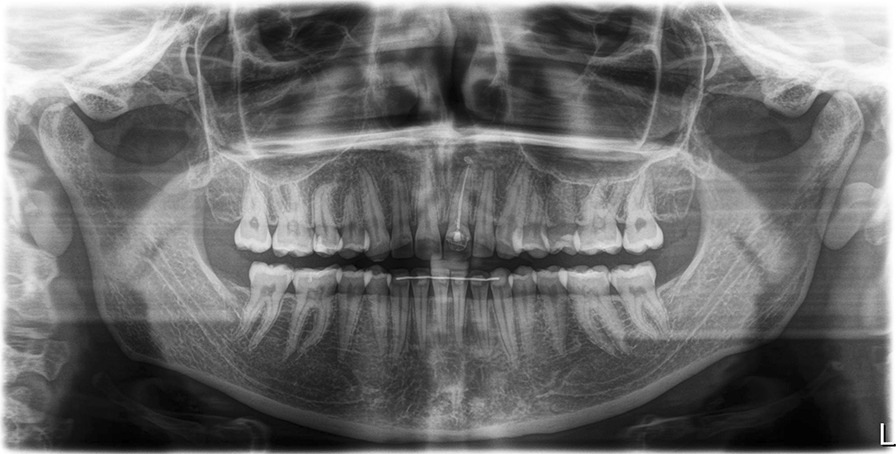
Panoramic radiograph of the patient showing symmetric but flattened condyles with a slightly widened TMJ gap on both sides
